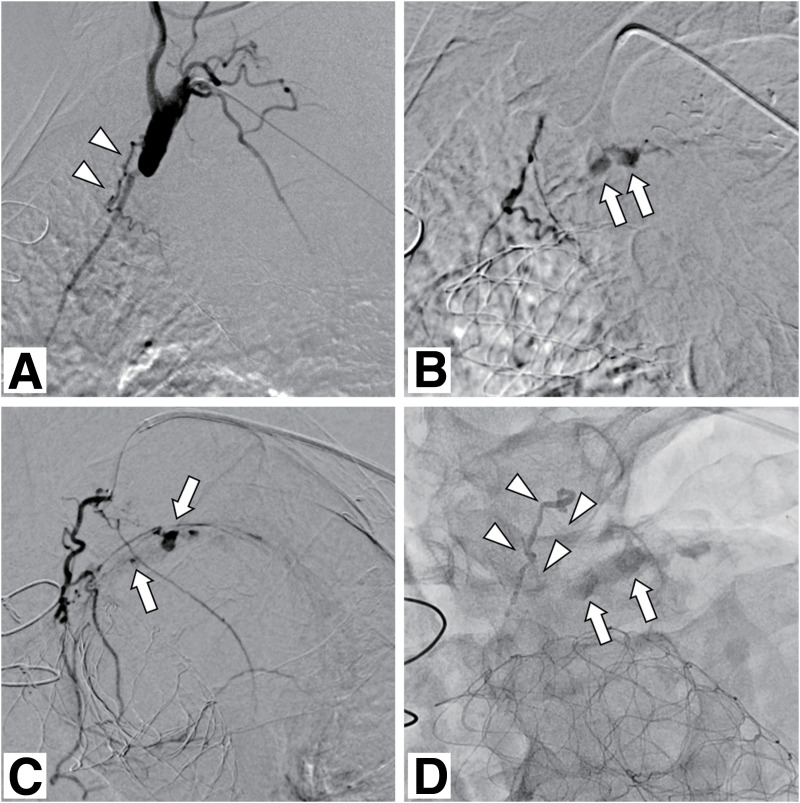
Endovascular treatment. (A) Angiography of the left subclavian artery demonstrates a small mediastinal branch corresponding to the findings on contrast-enhanced CT (arrowheads). (B, C) Selective angiography of the target mediastinal branch in the left anterior oblique (B) and anterior (C) views shows contrast enhancement within the aneurysm sac via presumed vasa vasorum originating from the distal portion of a small proximally branching vessel. Arrows indicate the endoleak. (D) A fluoroscopic image after embolization shows an NBCA cast within the aneurysm sac (arrows) and the target artery (arrowheads). CT: computed tomography; NBCA: N-butyl-2-cyanoacrylate
